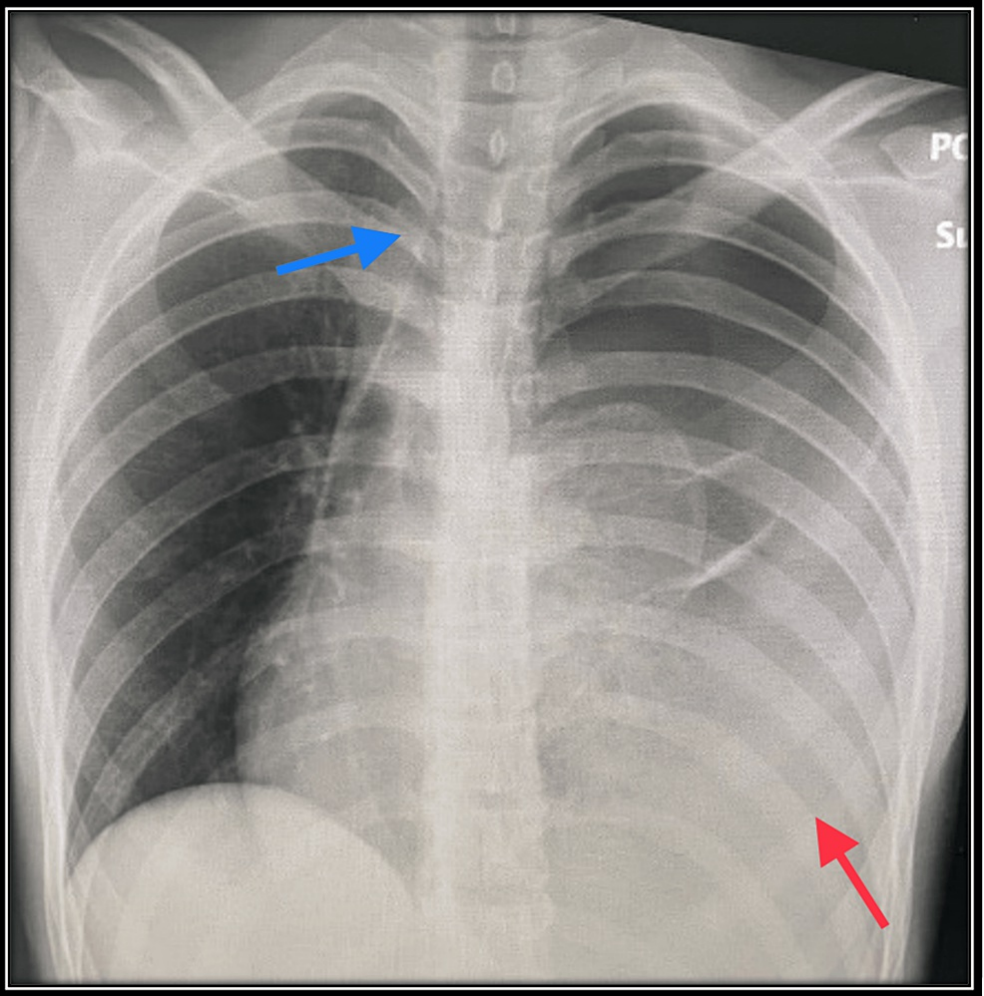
Chest X-ray of the patient on arrival at ER. The radiographic findings of a supine chest X-ray revealed a left-sided primary spontaneous pneumothorax and hemothorax (red arrow) with tracheal deviation to the contralateral side (blue arrow).
